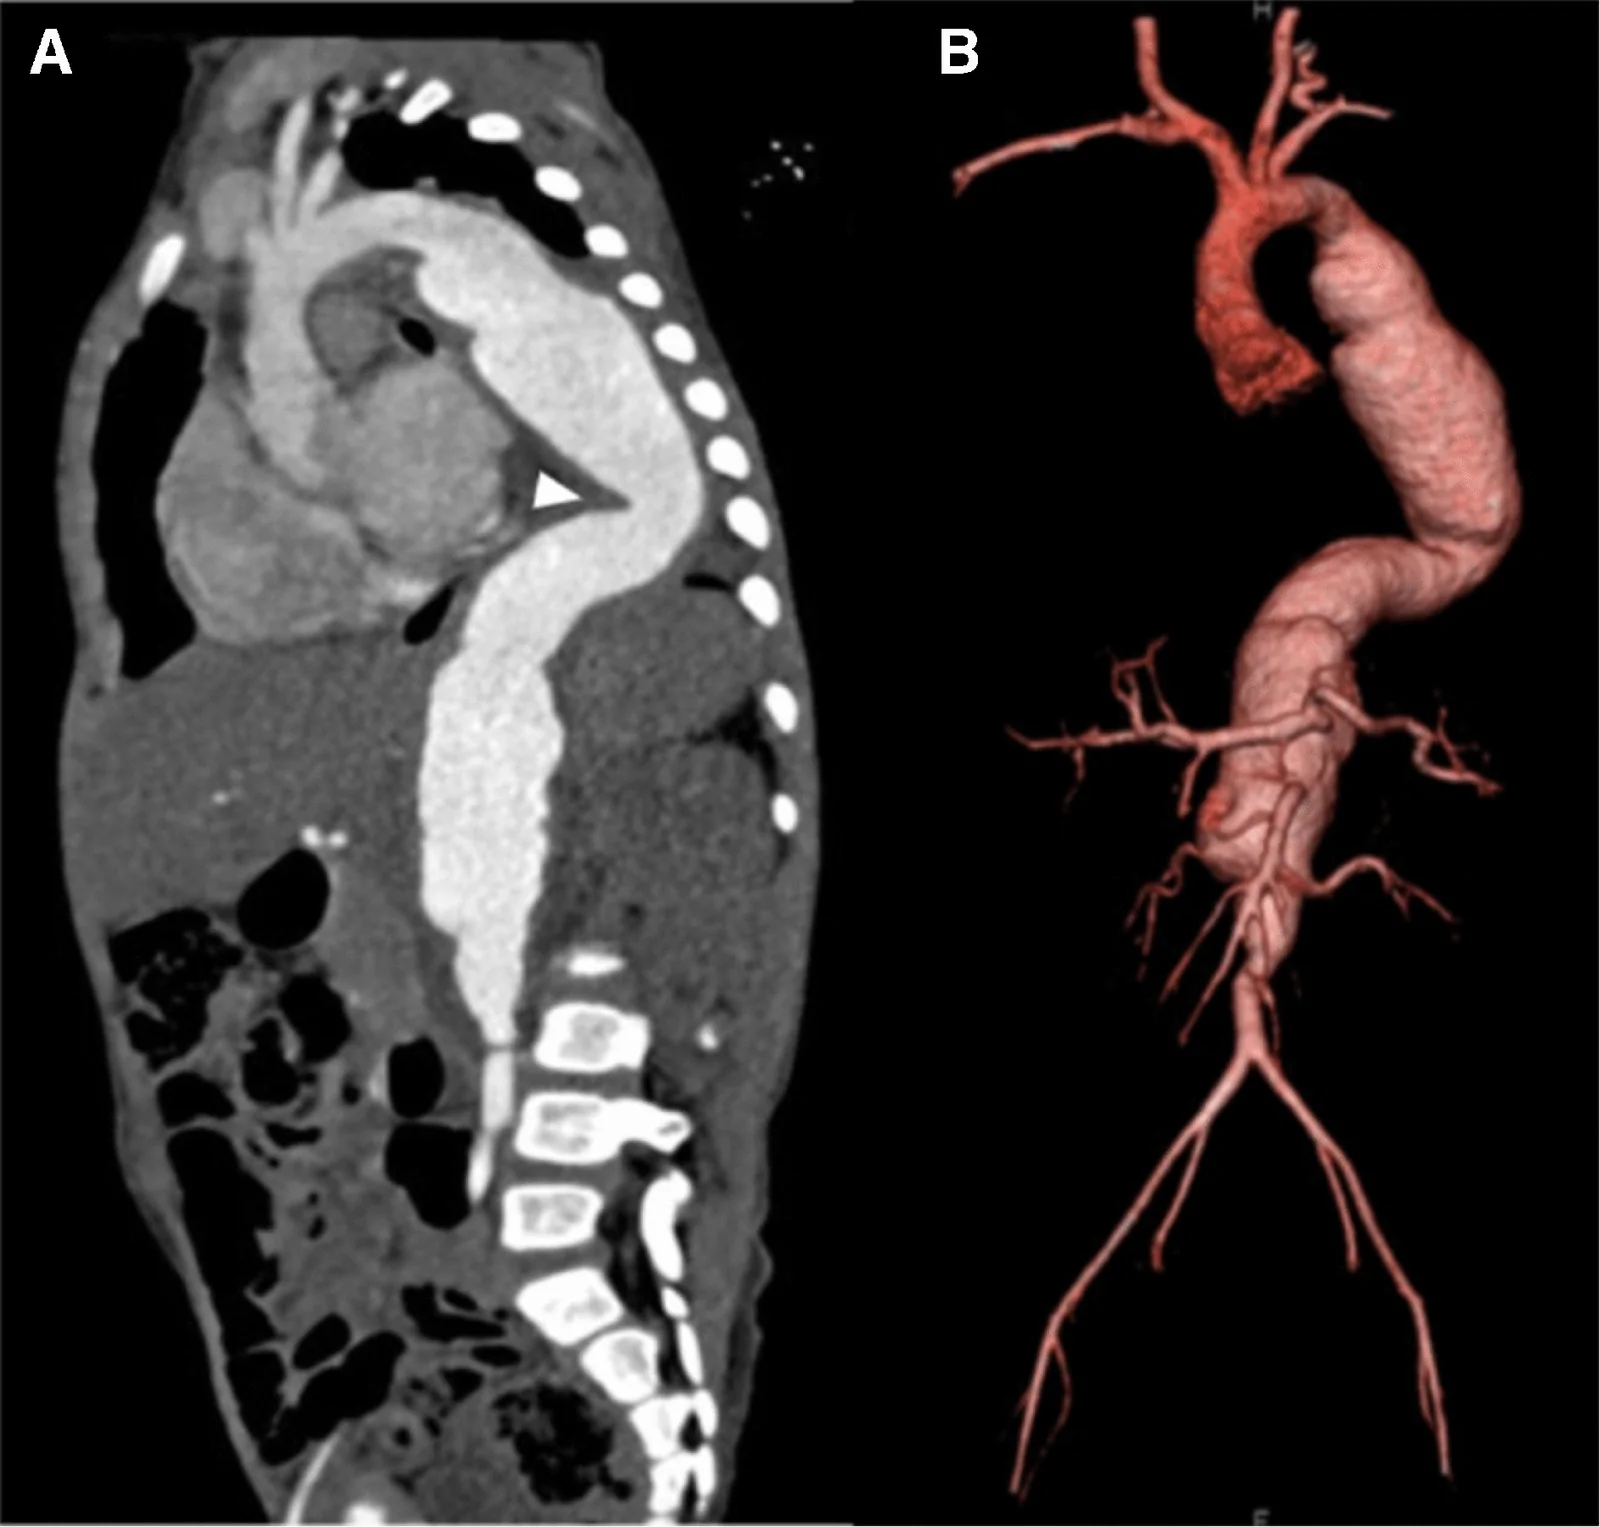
A 2-year-old girl with Takayasu arteritis. (A) Sagittal CT angiography reconstruction demonstrates a markedly dilated and tortuous thoracoabdominal aorta with focal kinking at the thoracoabdominal transition (arrowhead) (B) Three-dimensional volume-rendered CT angiography image shows irregular aneurysmal dilatation of the descending thoracic and abdominal aorta extending to the infrarenal segment.
